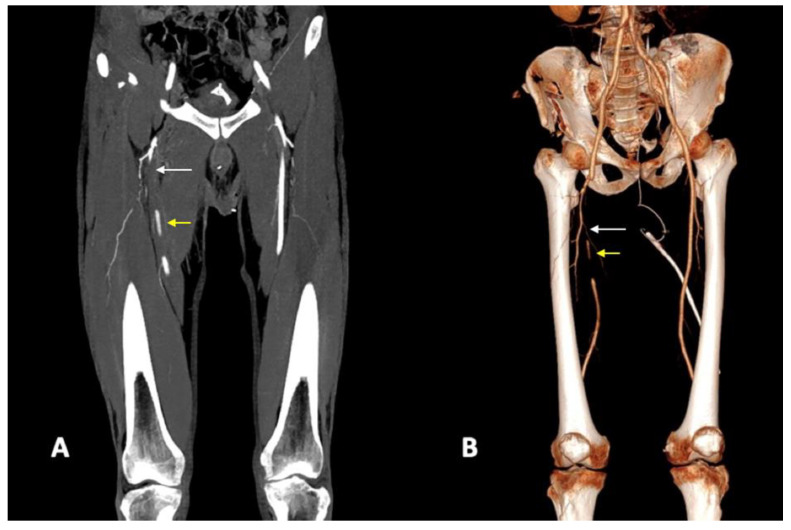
CTA, coronal planes, MIP (A), and 3D reconstructions (B). Arterial transections of proximal and medium tracts of right superficial femoral artery. In this patient, one may note both the complete loss of opacification of the proximal tract (white arrows) and the lower opacification of the downstream revascularized tract (yellow arrows) of the right superficial femoral artery, with reduced luminal caliber (narrowing).
